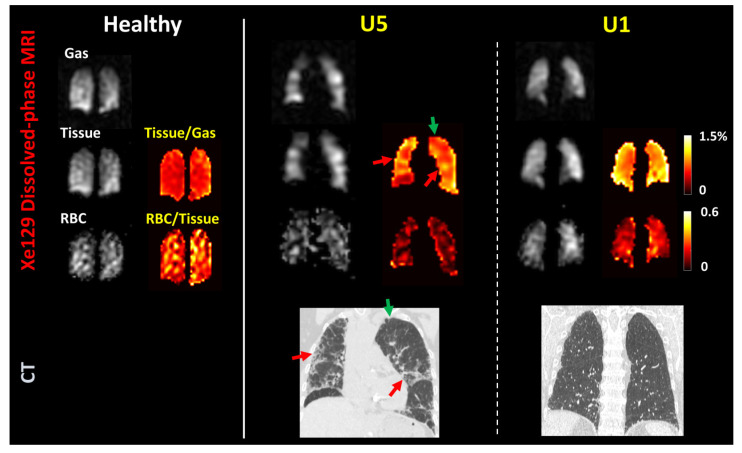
Representative dissolved-phase ^129^Xe MRI (top) and coronal high-resolution CT (bottom) results from one healthy subject (52-year-old woman, FVC 113% predicted, DLCO 111% predicted) and two subjects with UIP (subject U5 in the supplemental table: 79-year-old woman, FVC 53% predicted, DLCO 31% predicted; subject U1 in the supplemental table: 73-year-old man, FVC 76% predicted, DLCO 61% predicted). “Gas,” ^129^Xe in the airspace; “Tissue,” ^129^Xe dissolved in the lung interstitium; “RBC,” ^129^Xe associated with red blood cells. “Tissue/gas” and “RBC/Tissue” panels show the calculated tissue-to-gas and RBC-to-tissue ratio maps for each subject. Red arrows show areas of more severe fibrosis on CT corresponding to areas of increased tissue-to-gas ratios, and green arrow indicate areas of relatively normal lung on CT corresponding to areas of close to normal tissue-to-gas ratios.
